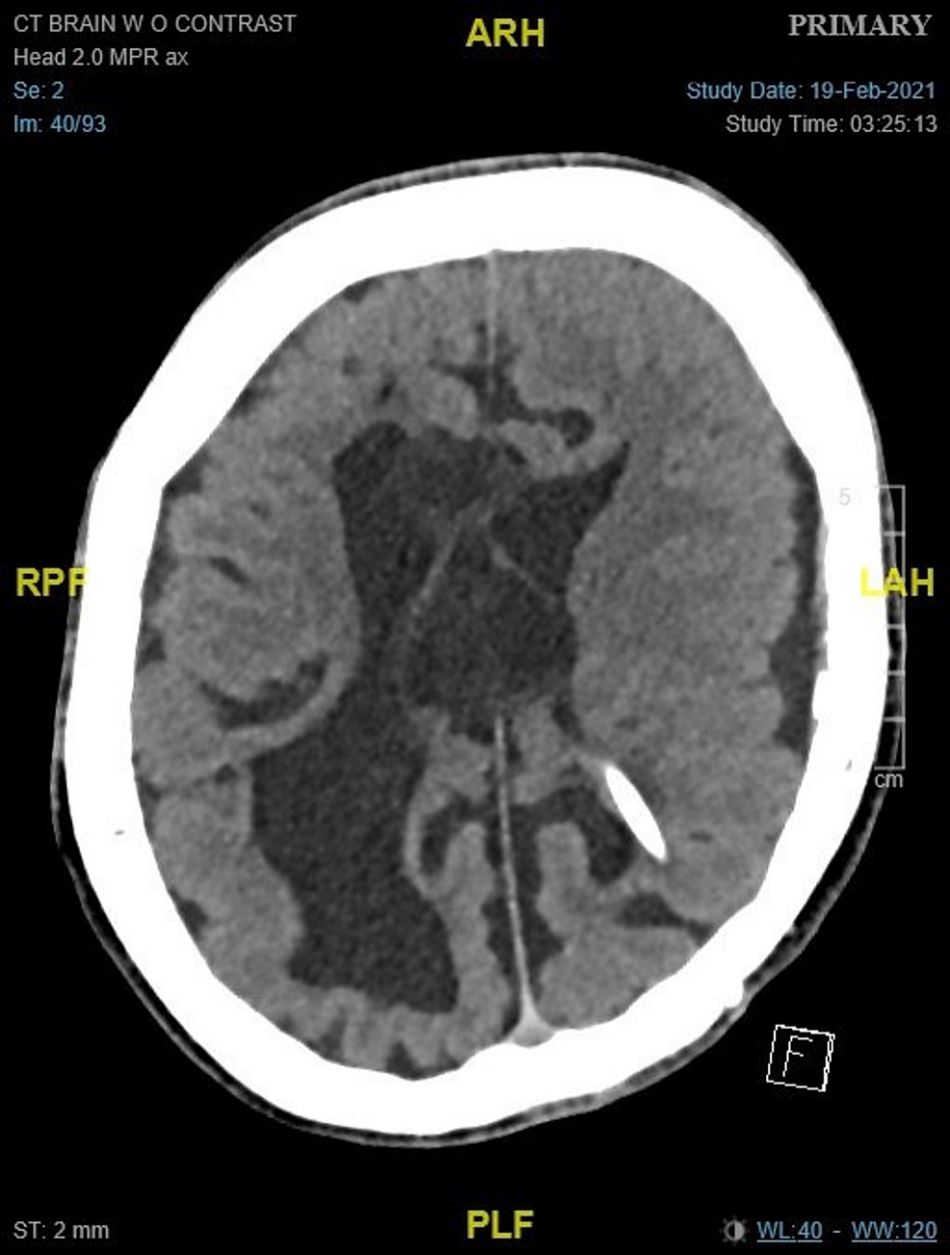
CT brain axial cut was done a few days before the patient's deterioration, showing the ventricular catheter in the left lateral ventricle and lax brain.
